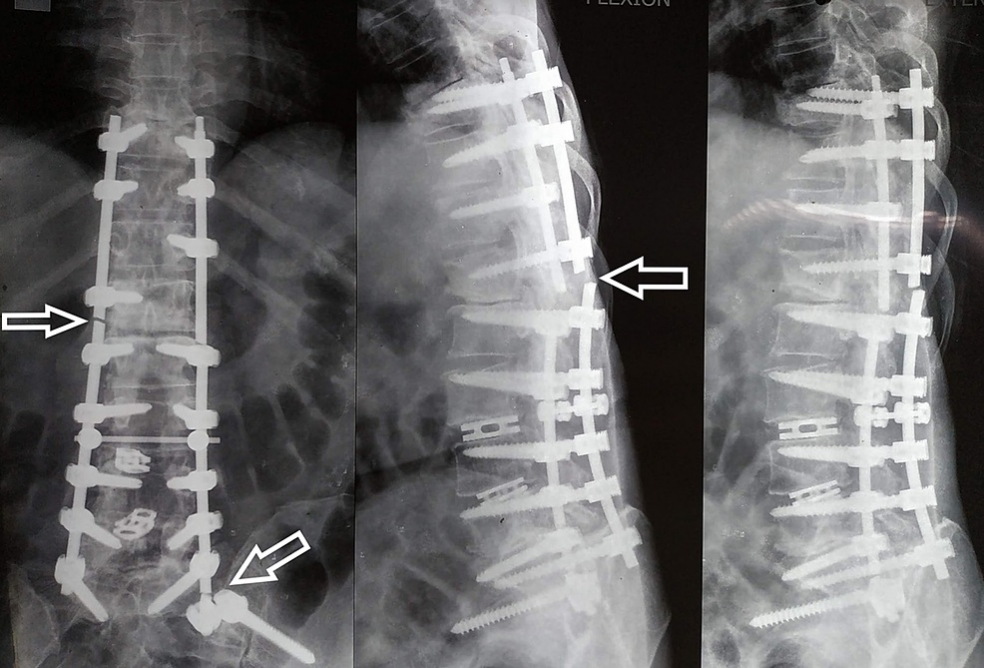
X-ray with anteroposterior and dynamic lateral views after five years of presentation with rod breakage
